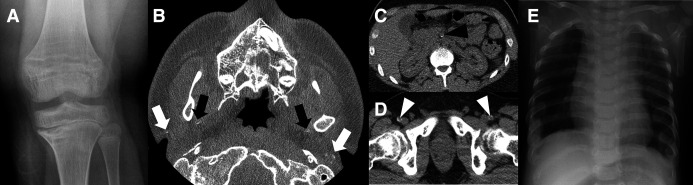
Radiological imaging supporting diagnosis of generalised arterial calcification of infancy in P4 (A–D) and Jeune thoracic dystrophy in P8 (E). (A) Anteroposterior radiograph of left knee aged 12 years demonstrating ‘wide epiphyseal plates of the knee’ with ‘frayed metaphyses of the femora’ and ‘frayed metaphyses of the tibiae’, the latter affecting the medial aspect of the proximal tibia. Appearances are consistent with hypophosphataemic rickets. (B) Axial image from dental CT examination performed aged 20 years. There is mural calcification of the facial arteries (black arrows) and calcification of the included portion of the pinnae of the ears (white arrows). (C) and (D) Axial images from non-contrast urinary tract CT examination aged 20 years. There is mural calcification of the superior mesenteric artery (black arrowhead in C) and both common femoral arteries (white arrowheads in D). Increased density is noted in the renal medullae (C), consistent with early medullary nephrocalcinosis. Calcification of hepatic artery branches was also noted (not shown). In addition, calcification of the popliteal arteries was noted on a CT examination of the lower limbs at age 14 years (not shown). (E) Anteroposterior chest radiograph (aged 6 months) shows a ‘narrow chest’ with ‘short ribs’ and ‘prominent ribs’'. Images annotated using terms from the dREAMS ontology (https://d-reams.org) in inverted commas.
